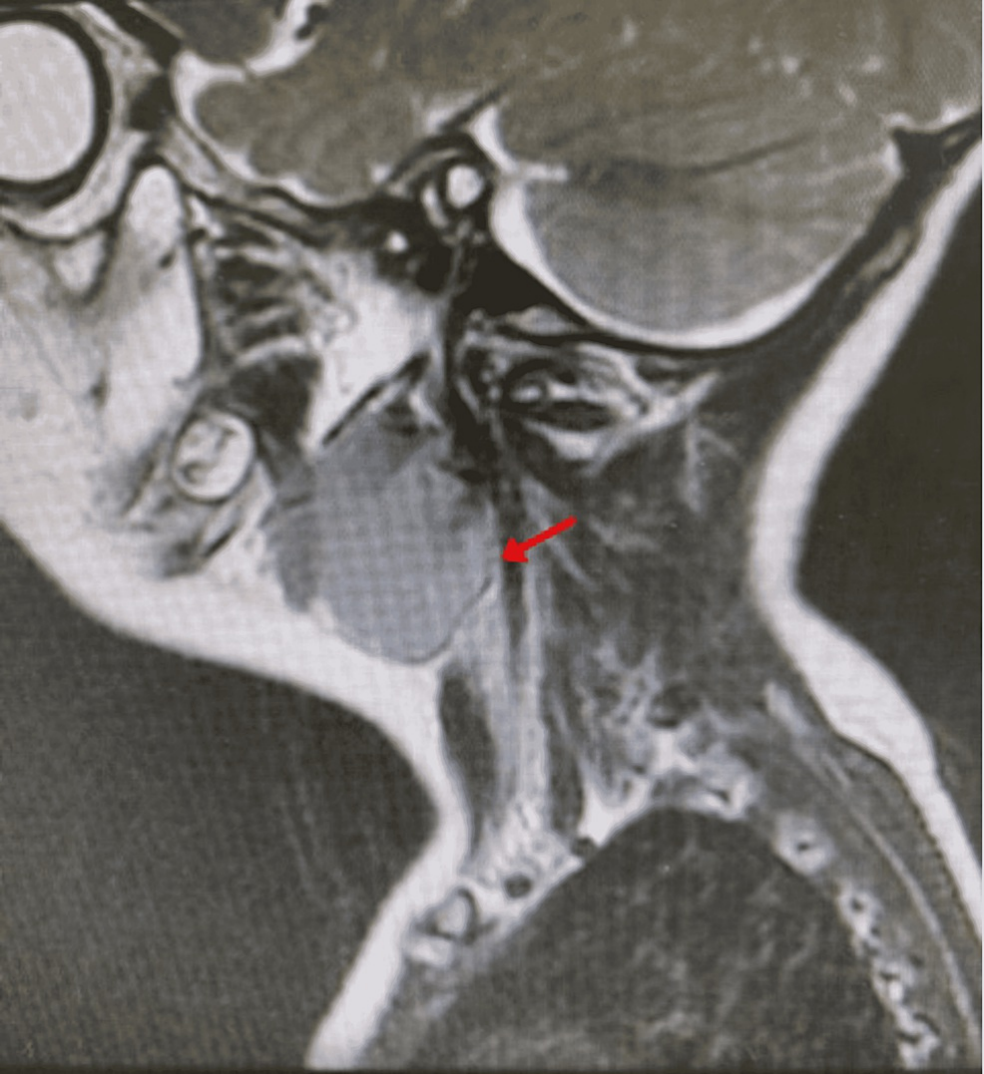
T2 head and neck MRI in sagittal view showing isointense mass in the submandibular region suggesting ectopic thymus (red arrow).
